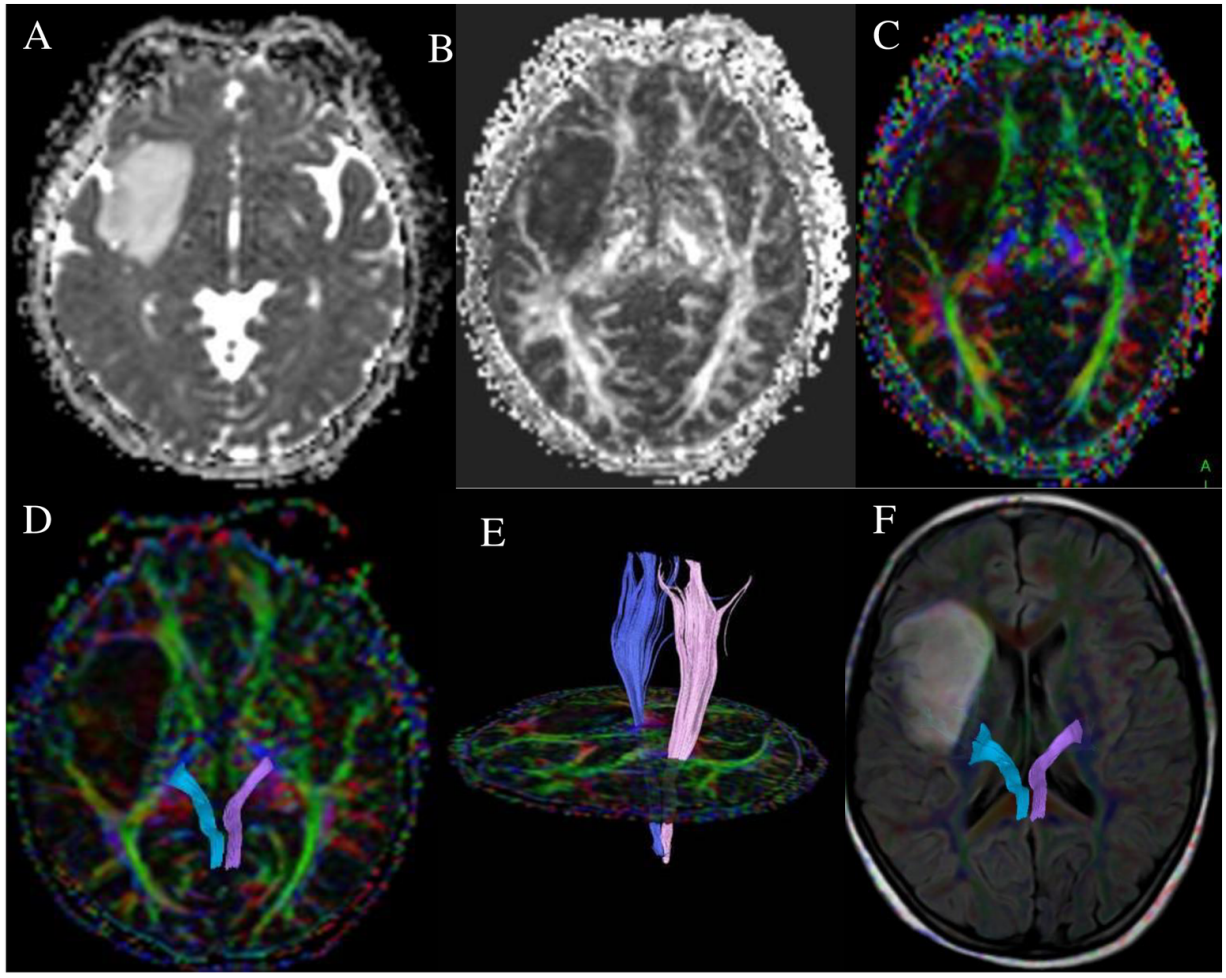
A 40-year-old female with right frontotemporal astrocytoma (WHO grade II) and left lower limb muscle strength was MMT 5. ADC map (A) show the solid part of tumor was slightly lower signal, FA map (B) and color encoding pattern map (C) and DTT map (D, E) on the right side of the corticospinal tract showed mild displacement, DTT-FLAIR show around of the CST without edema.
